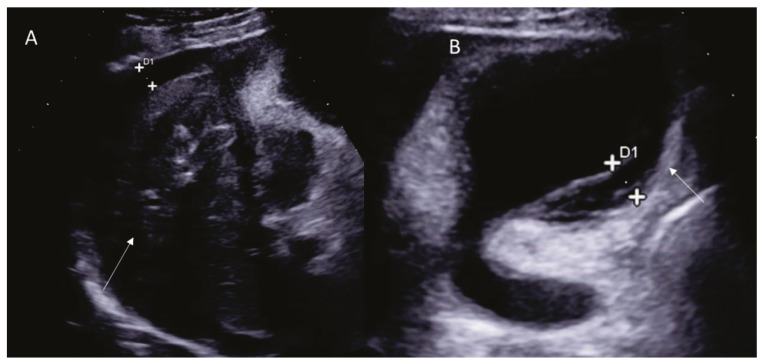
Woman 58 year at 1-week follow-up after MWA of liver metastasis. MRI assessment US assessment of RFA treated HCC on V segment ((A); arrow). In (B) arrow shows cholecystitis.
